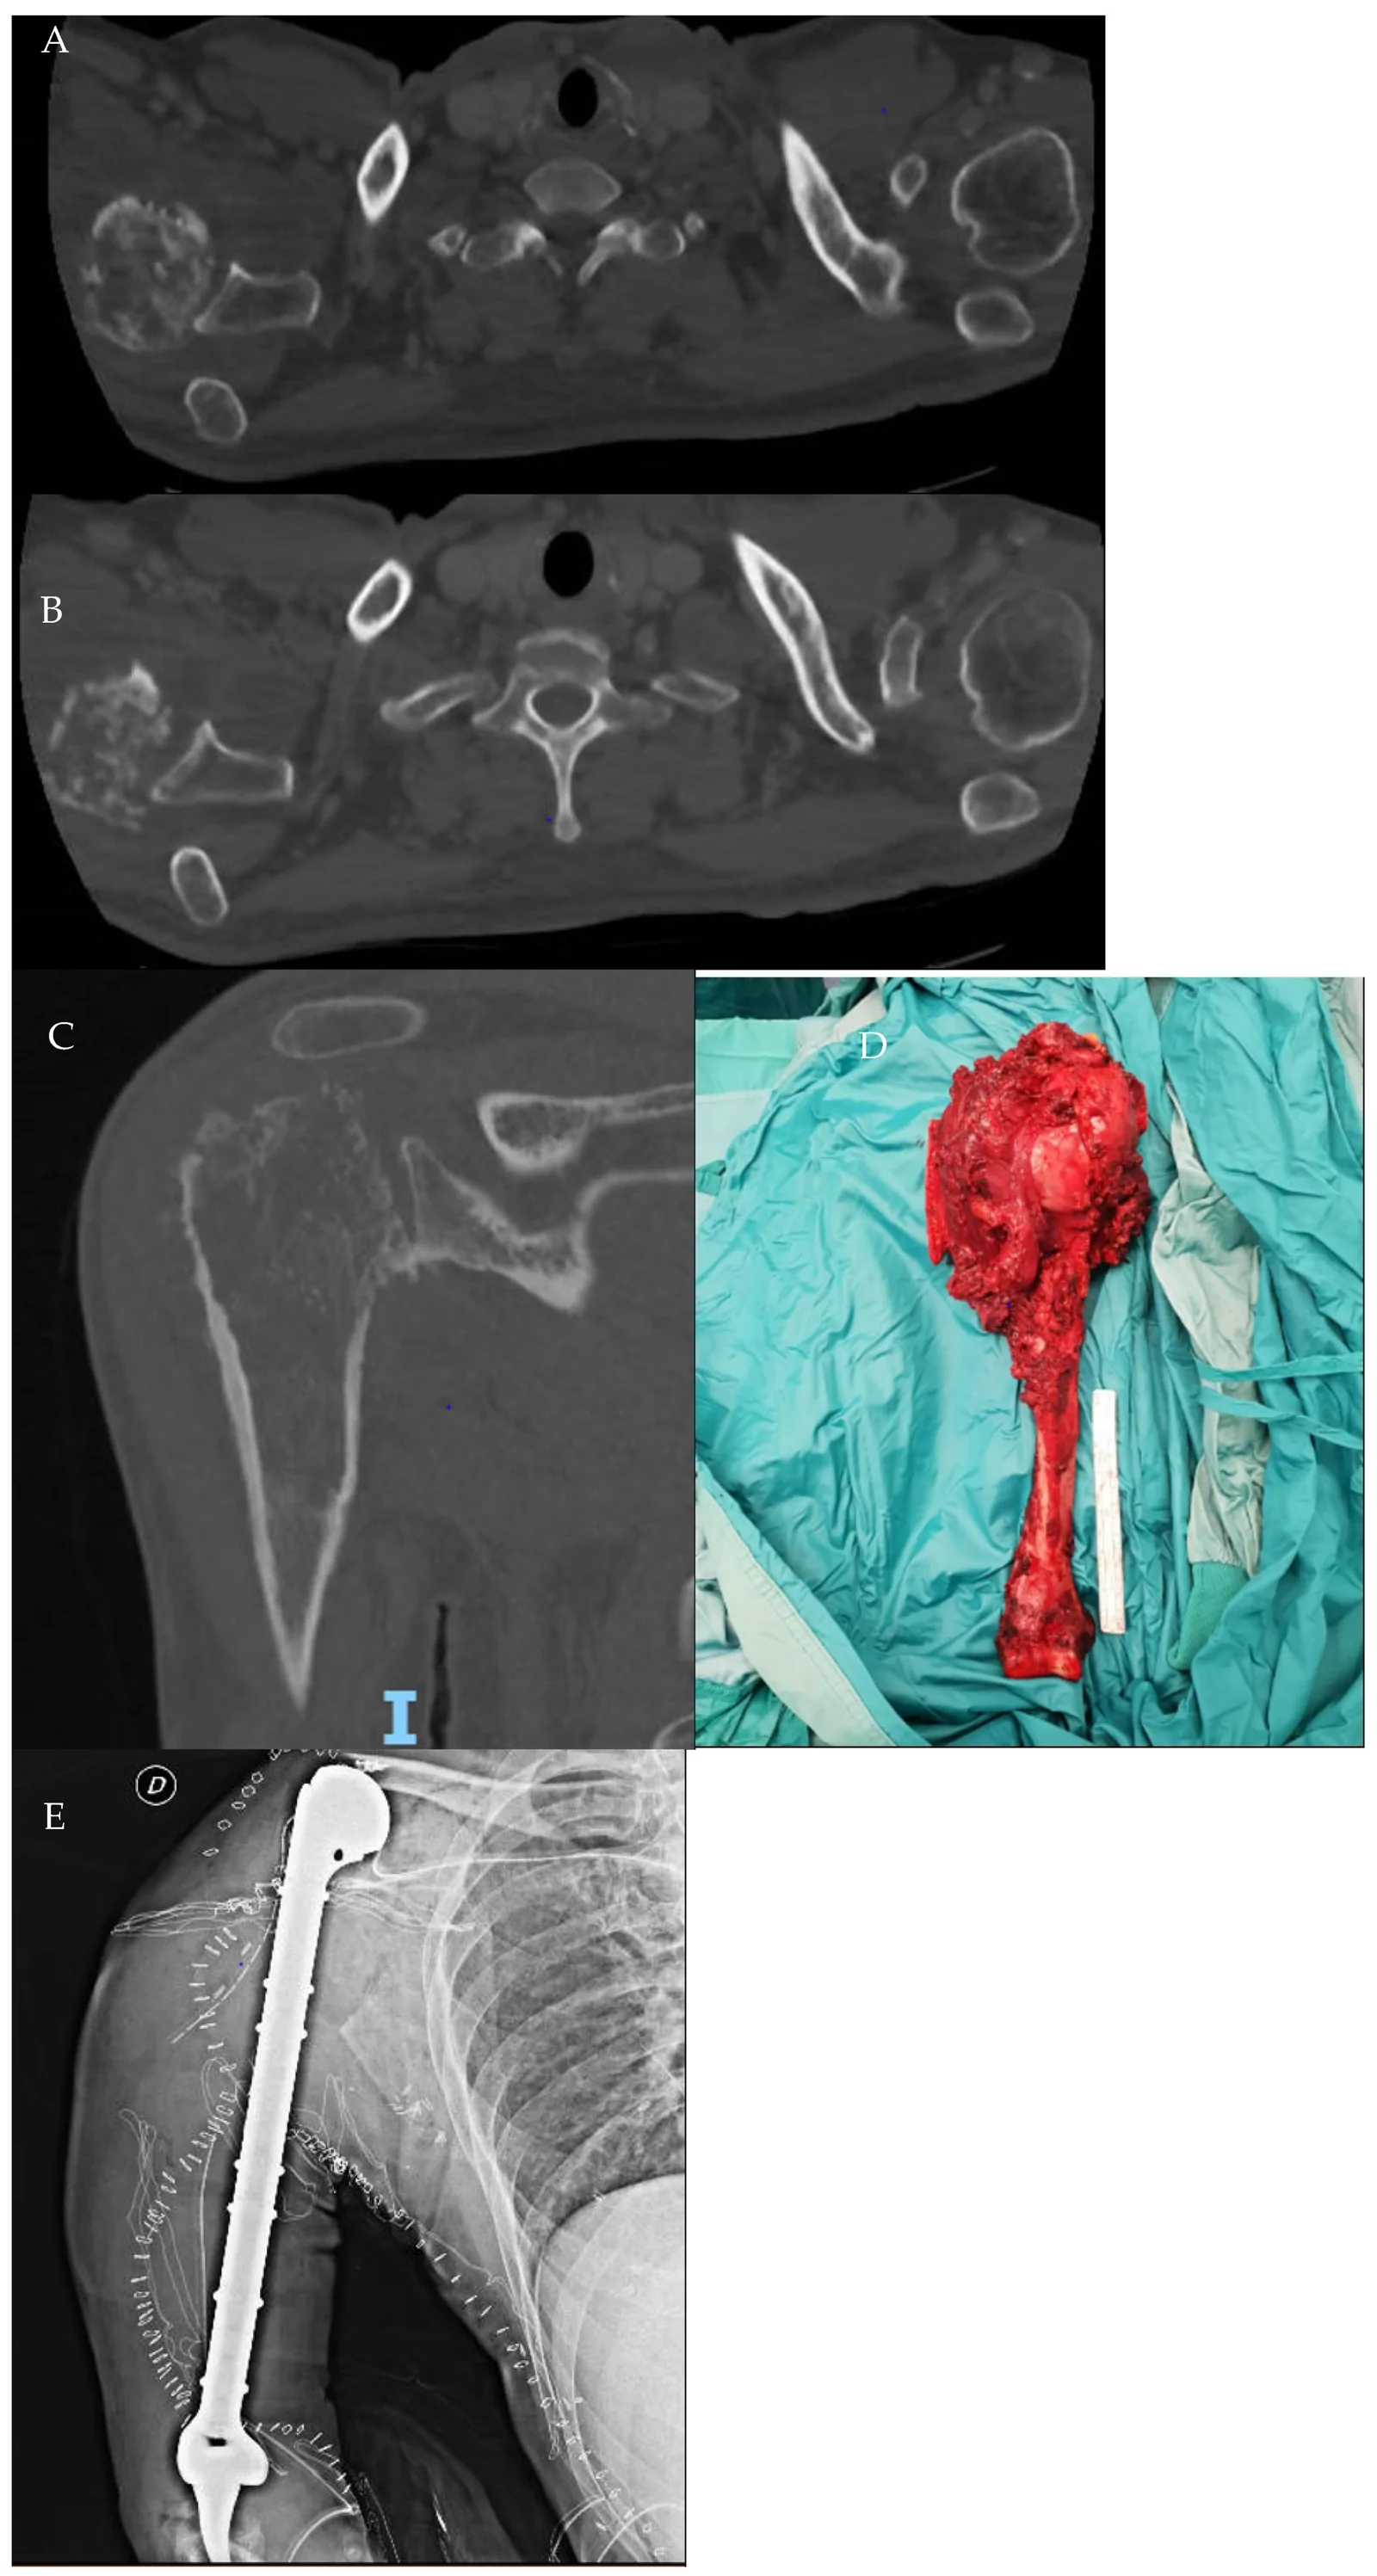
(A,B) Axial CT scan views; (C) coronal CT scan view; (D) total humerus resection for chondrosarcoma in a patient undergoing extra-articular resection; (E) postoperative X-ray showing total humerus replacement with an endoprosthesis in a patient treated with a latissimus dorsi flap.
